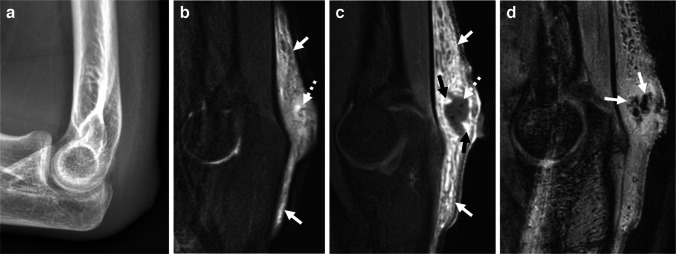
Septic bursitis. A 17-year-old male presented with cellulitis and septic olecranon bursitis after getting a tattoo. a Lateral radiograph of the elbow shows soft tissue swelling overlying the olecranon with reticular subcutaneous edema. Sagittal T2W FS (b) and sagittal T1W FS post-contrast (c) MR images show extensive T2 hyperintense reticular signal which enhances on the post-contrast image (*solid white arrows*), consistent with cellulitis. Additionally, there is a focal heterogenous collection at the location of the olecranon bursa that shows central non-enhancement (*dashed arrows*) and nodular peripheral hyperenhancement (*black arrows* in c) consistent with septic bursitis. d Sagittal gradient echo image shows central foci of blooming artifact (*arrows*) indicative of gas. There was no concurrent septic arthritis. Incision and drainage were performed and cultures were positive for oxacillin-resistant *Staphylococcus aureus*. Symptoms resolved after drainage and antibiotics
